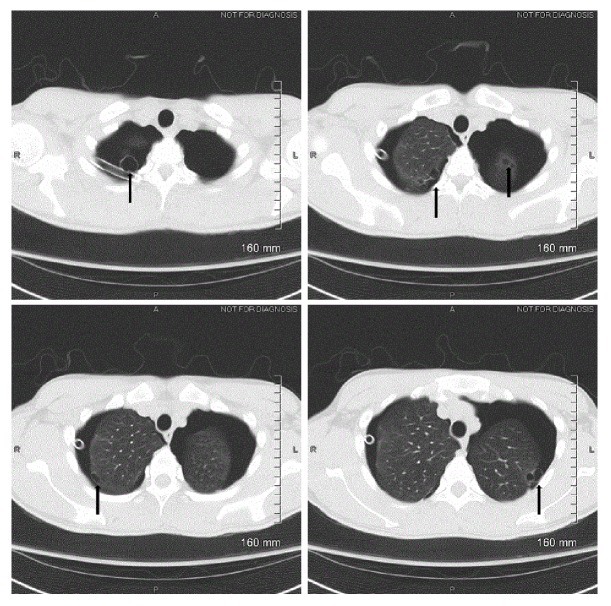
Chest computed tomography scan showing multiple subpleural blebs (arrow head).
